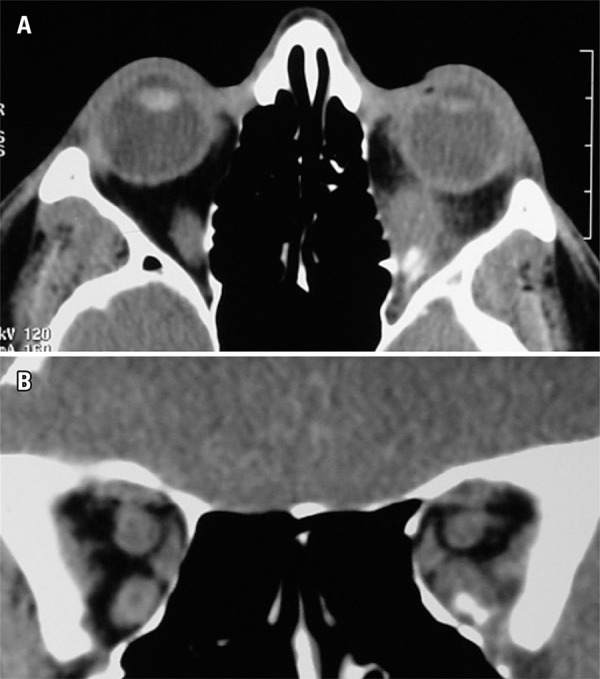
Computed tomography scans of the orbits show enlarged left inferior rectus muscle with an infiltrated and calcified lesion. (A) Axial view; (B) Coronal view
